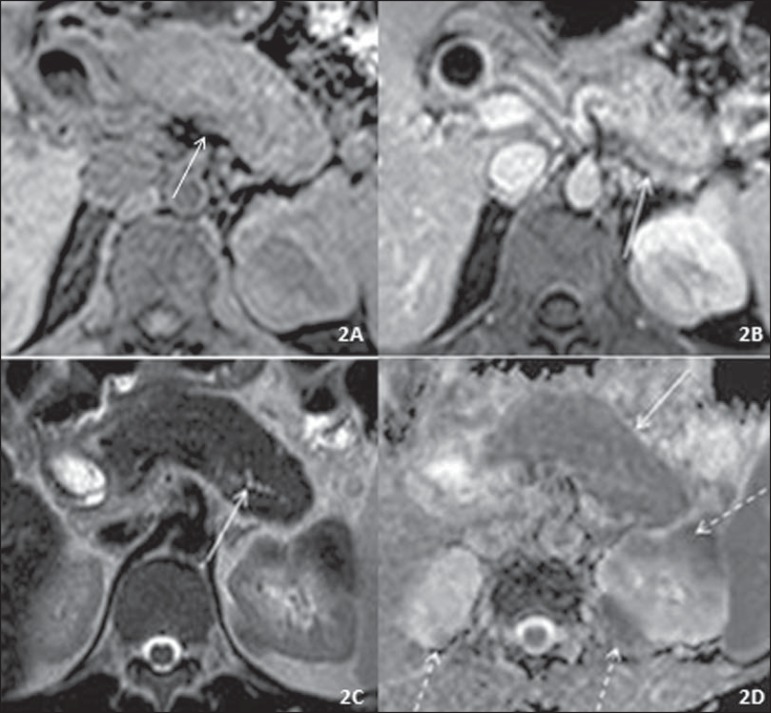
Axial abdominal MRI scan. A: T1-weighted image without
